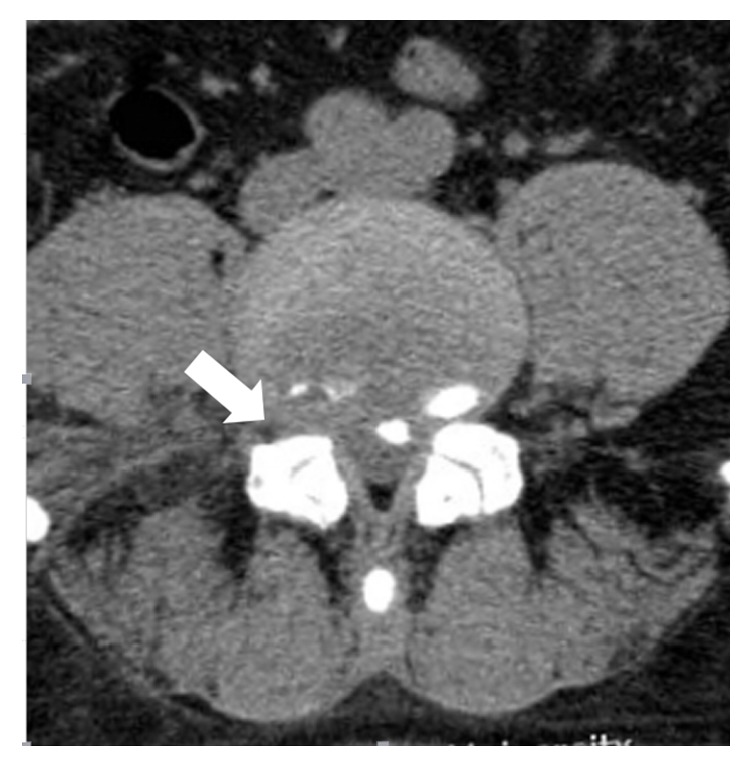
Postop axial CT image (white arrow) shows enlargement of narrowed foramen (compared to contralateral side) and preservement of the facet joint.
